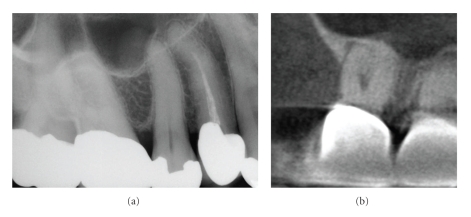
Difficulty in visualizing furcal and apical lesions with periapical radiography due to lack of coverage, anatomic superimposition, and geometric distortion is well established. The periapical radiograph (a) of the maxillary right second molar in an asymptomatic patient is unremarkable; however there is lack of coverage of the second molar posteriorly and marked superimposition of the distal root of the first molar over the mesial root of the second molar. The corresponding cropped sagittal CBCT image (b) of the second molar demonstrates a furcal radiolucency and associated periradicular periodontitis. Subsequent clinical investigation found this tooth to be nonvital.
